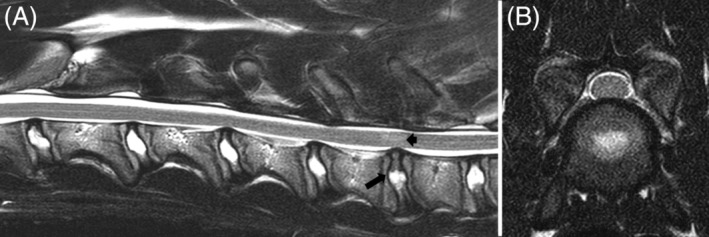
Magnetic resonance imaging of a 7‐month‐old Great Dane with cervical spondylomyelopathy because of intervertebral disk protrusion. A, Sagittal T2‐weighted image showing spinal cord compression at C6‐C7 because of intervertebral disk protrusion (long arrow). Note the partial degeneration of intervertebral disks at C2‐C3, C5‐C6, C6‐C7, C7‐T1. There is also hyperintensity of the spinal cord parenchyma at C6‐C7 (short arrow). B, Transverse T2‐weighted image showing intervertebral disk protrusion causing mild spinal cord compression at C6‐C7
